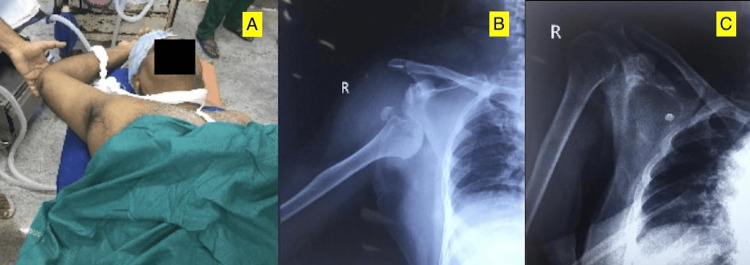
Case 3 A) The right arm and shoulder are in a hyper-abducted position with inferior shoulder fracture-dislocation. B) Radiograph showing right sub-glenoid inferior shoulder fracture-dislocation. C) Post-reduction radiograph showing shoulder in situ after 'two-step manoeuvre'.
